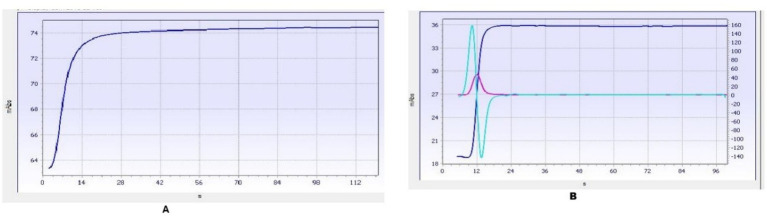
Clotting curve analysis of the patient. (A) Patient’s FIB clotting curve (Clauss method) shows fibrin formation but lacking a plateau phase, with ΔmAbs below the instrument threshold (15 mAU), resulting in a reported value of <0.35 g/L. The clotting time is paradoxically shortened. A normal control curve is shown for comparison, demonstrating a clear plateau phase and adequate amplitude. (B) Patient’s PT-derived FIB clotting curve showing morphological similarity to a low FIB control curve, with reduced amplitude and plateau. CT, clotting time; ΔmAbs, change in milli-absorbance units; mAU, milli-absorbance units; Th, threshold.
